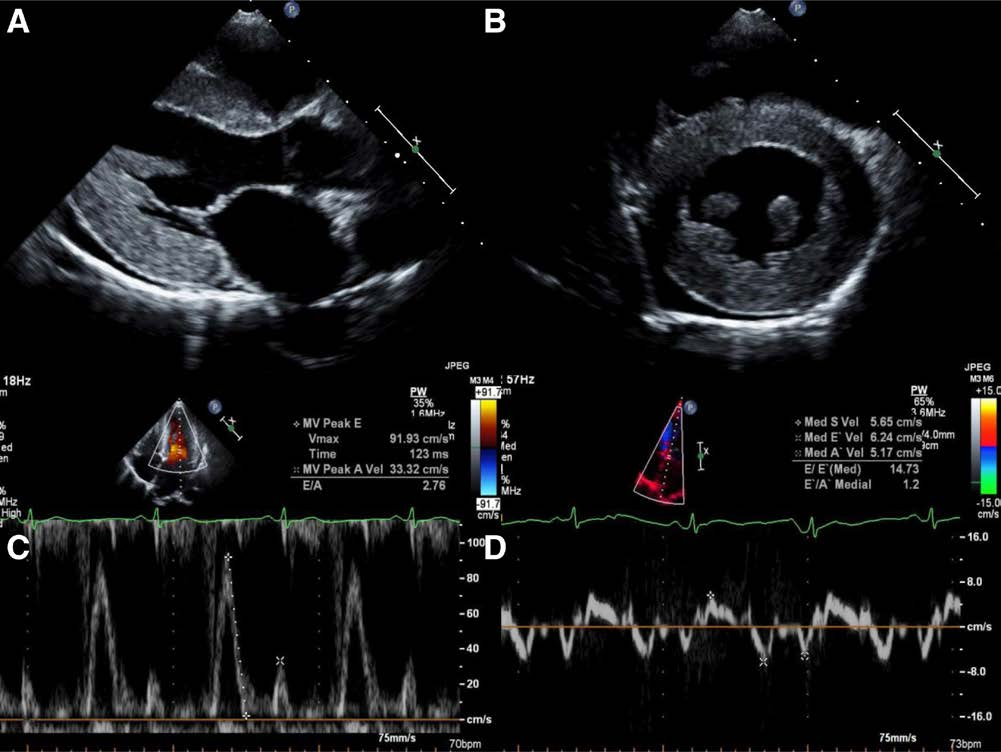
Transthoracic echocardiograms of Patient 1. (A) Parasternal long axis. (B) Parasternal short axis. (C) Mitral inflow. (D) Septal mitral annulus tissue Doppler image.
